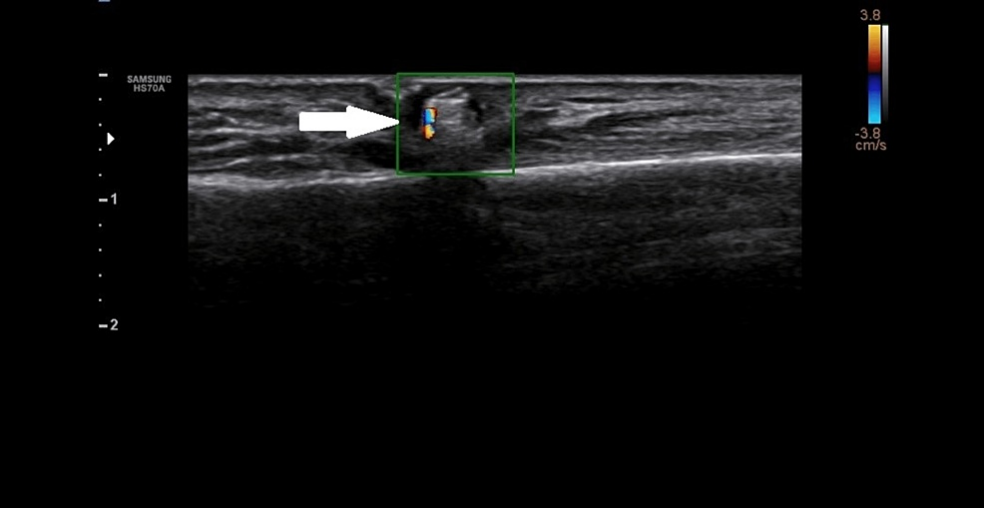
Colour Doppler image showing twinkling artifact (arrow)
